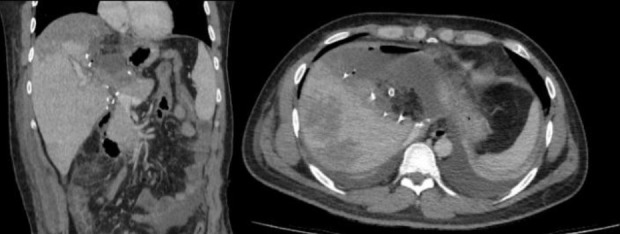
Post therapy CT scan indicating complete resection of the tumor with no evidence of remnant/metastatic disease
